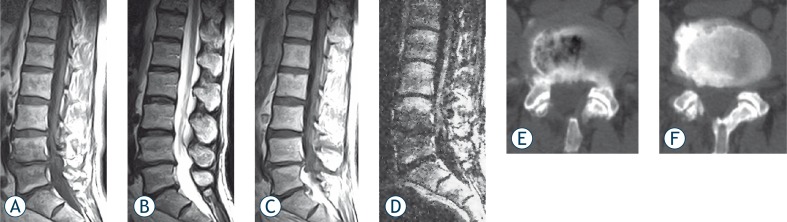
Sagittal MR images from 53 years old male patient with low back pain. A) T1-weighted MR image demonstrating low-signal intensity changes adjacent to the L4–5 disk, and B) T2-weighted MR image of the same level demonstrating high-signal intensity changes. C) On post-contrast T1-weighted images end-plates disclose signal intensity increase, which can also be seen in spondylodiscitis. D) On DWI end-plates showing low signal intensity changes consistent with Modic type 1 change at L4 through L5. E) and F) Axial CT slices obtained from the L4–5 disk level showing discal vacuum phenomenon and sclerosis, supporting the diagnosis of degenerative disc disease.
